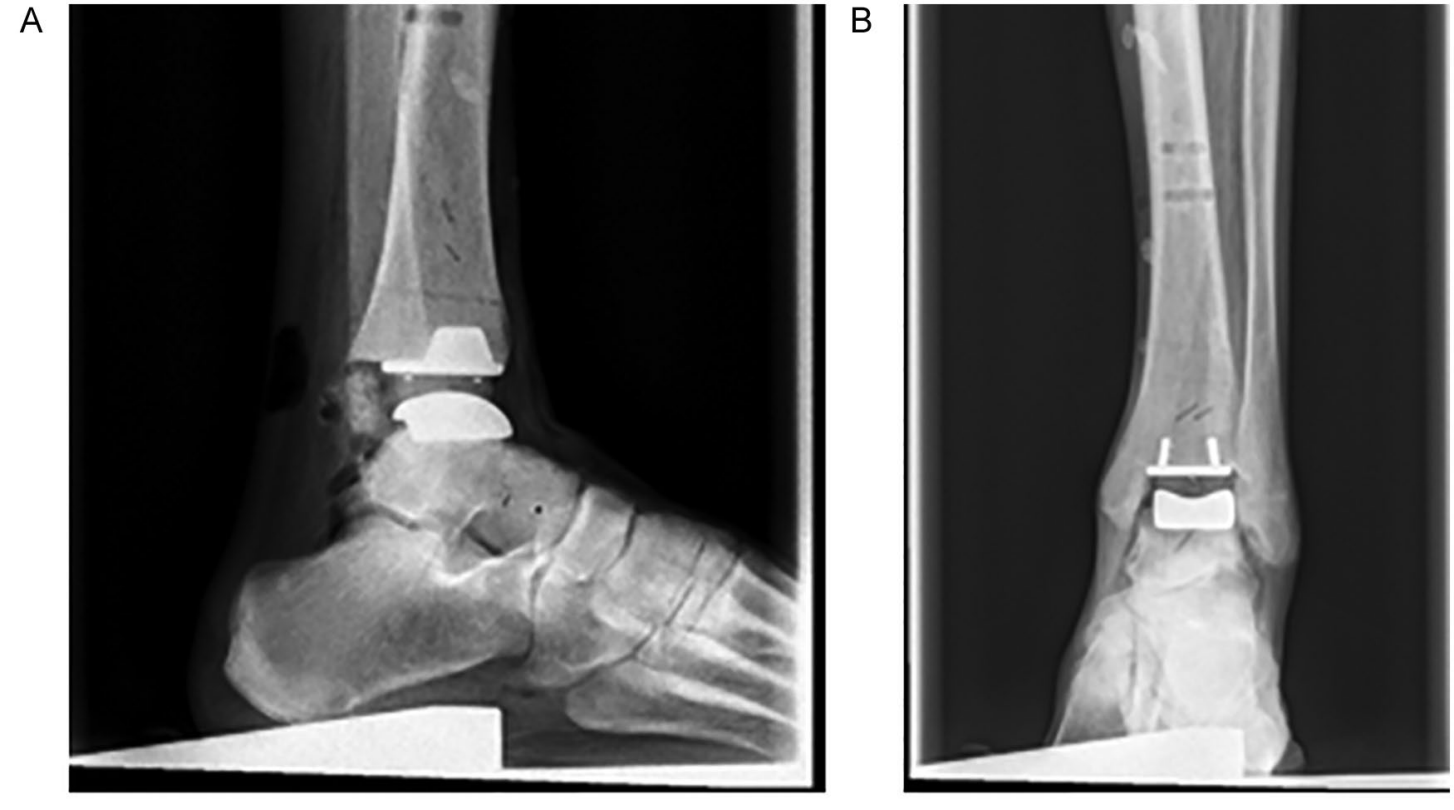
Assessment of prosthesis placement based on X-ray: A. Sagittal plane. B. Frontal plane. The prosthesis placement was assessed compared to the anatomical tibial axis.
